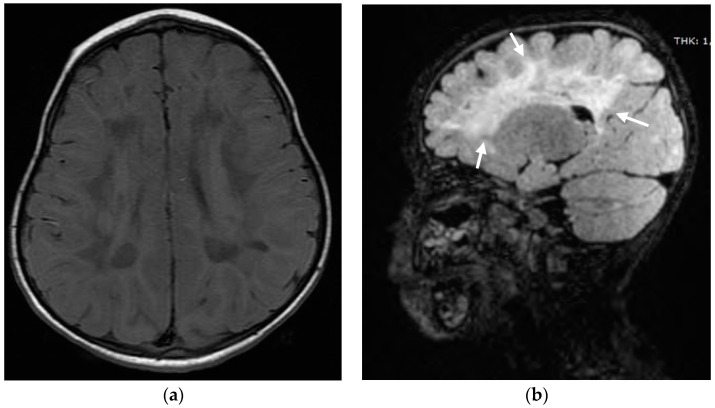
(a) Axial T1 weighted sequence. (b) Sagittal FLAIR cube weighted sequence, showing leukodystrophy (see white arrows).
